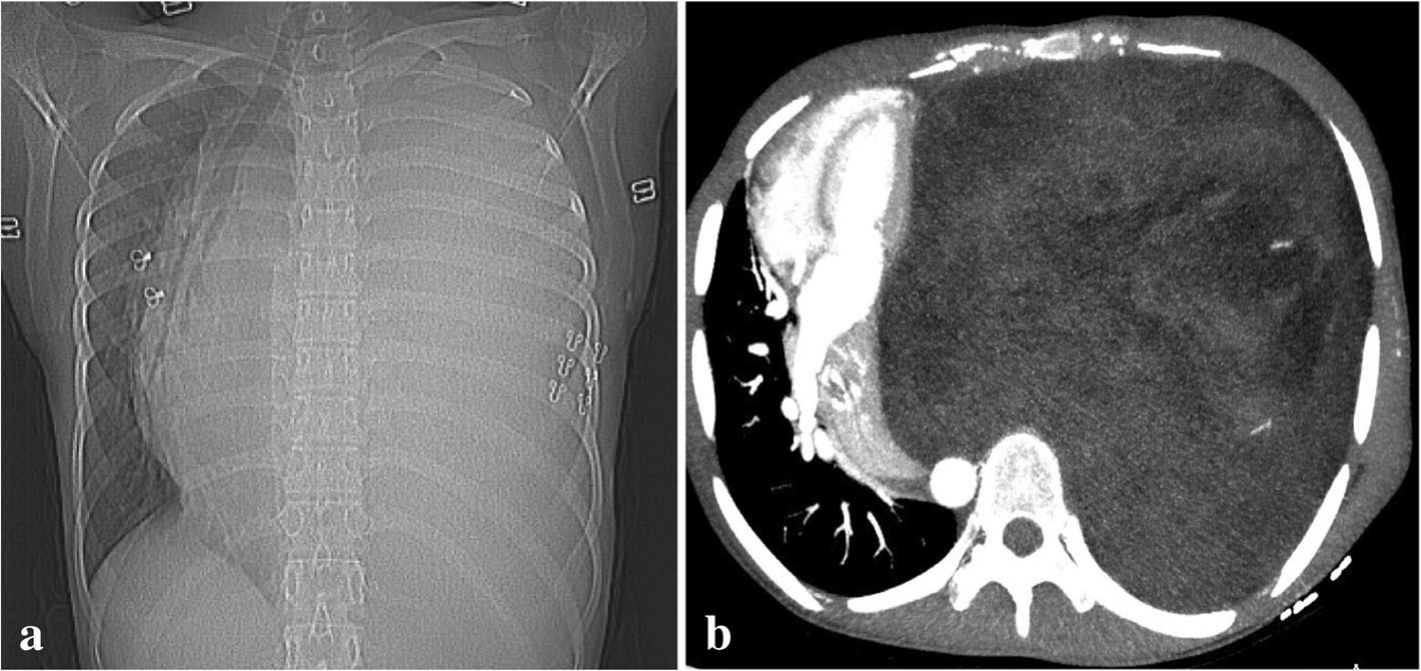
Computed tomography angiography images (a is sagittal image and b is cross-sectional image), giant mixed density masses, substantial compression of the left lung and heart
